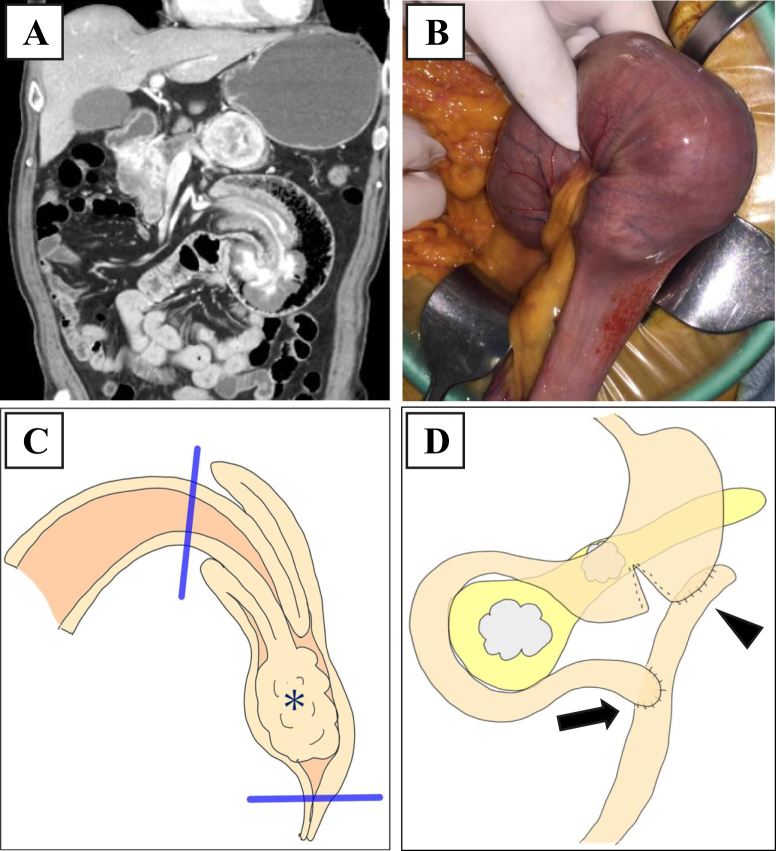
Operative findings. (A) Contrast-enhanced computed tomography demonstrating intussusception in the proximal jejunum. (B) Intraoperative finding showing a tumor originating from the ascending portion of the duodenum and extending into the jejunum. (C) Partial resection of the duodenojejunal junction including the tumor. * Tumor location; blue line indicates the resection line. (D) Bypass reconstruction with gastrojejunostomy (arrowhead) and duodenojejunostomy (arrow).
